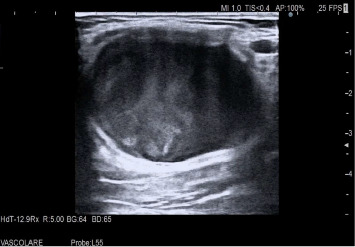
Color doppler ultrasound, “yin–yang” pattern.
